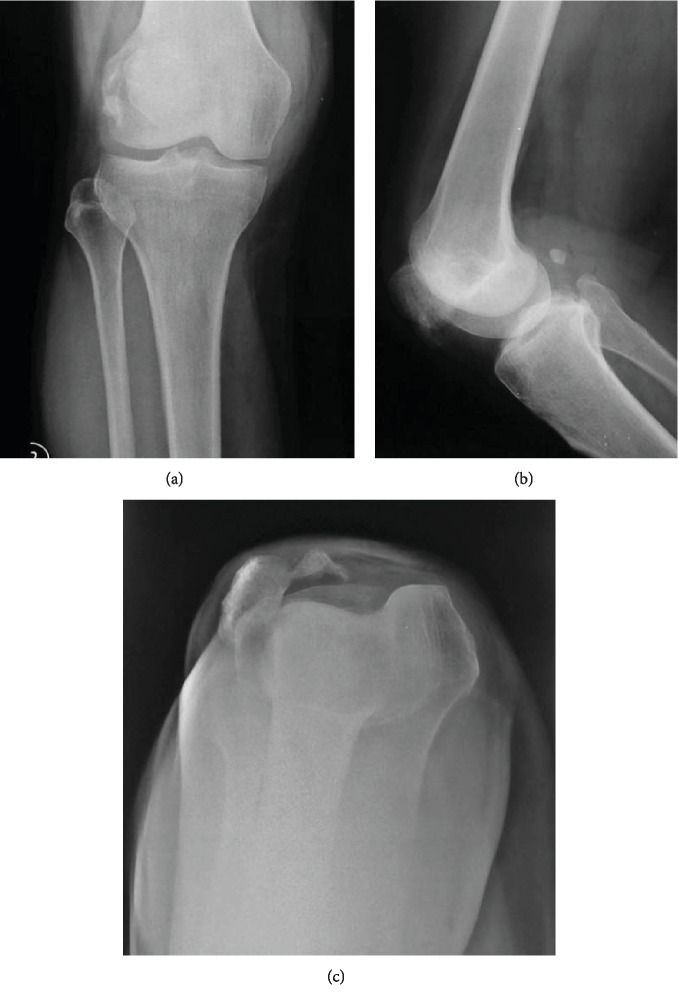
Radiographic imaging of the right knee in anteroposterior (a), lateral (b), and skyline (c) view showed lateral patellar dislocation.
